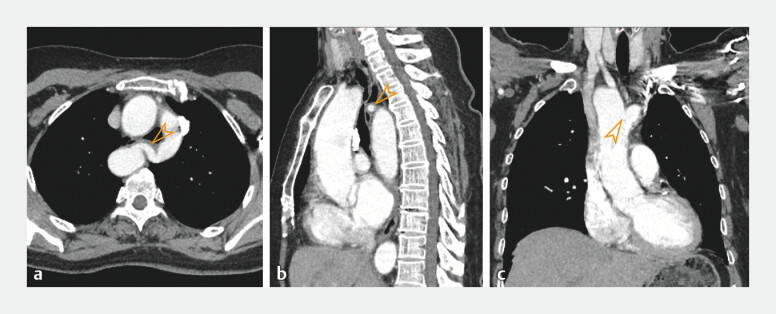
Computed tomography (CT) findings of the chest. Contrast-enhanced CT scanning demonstrated a vascular ring (arrowhead) compressing the esophagus posteriorly on:
